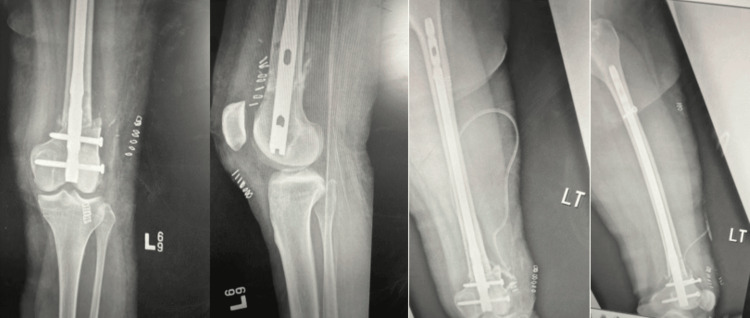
Case 4 immediate postoperative X-rays
